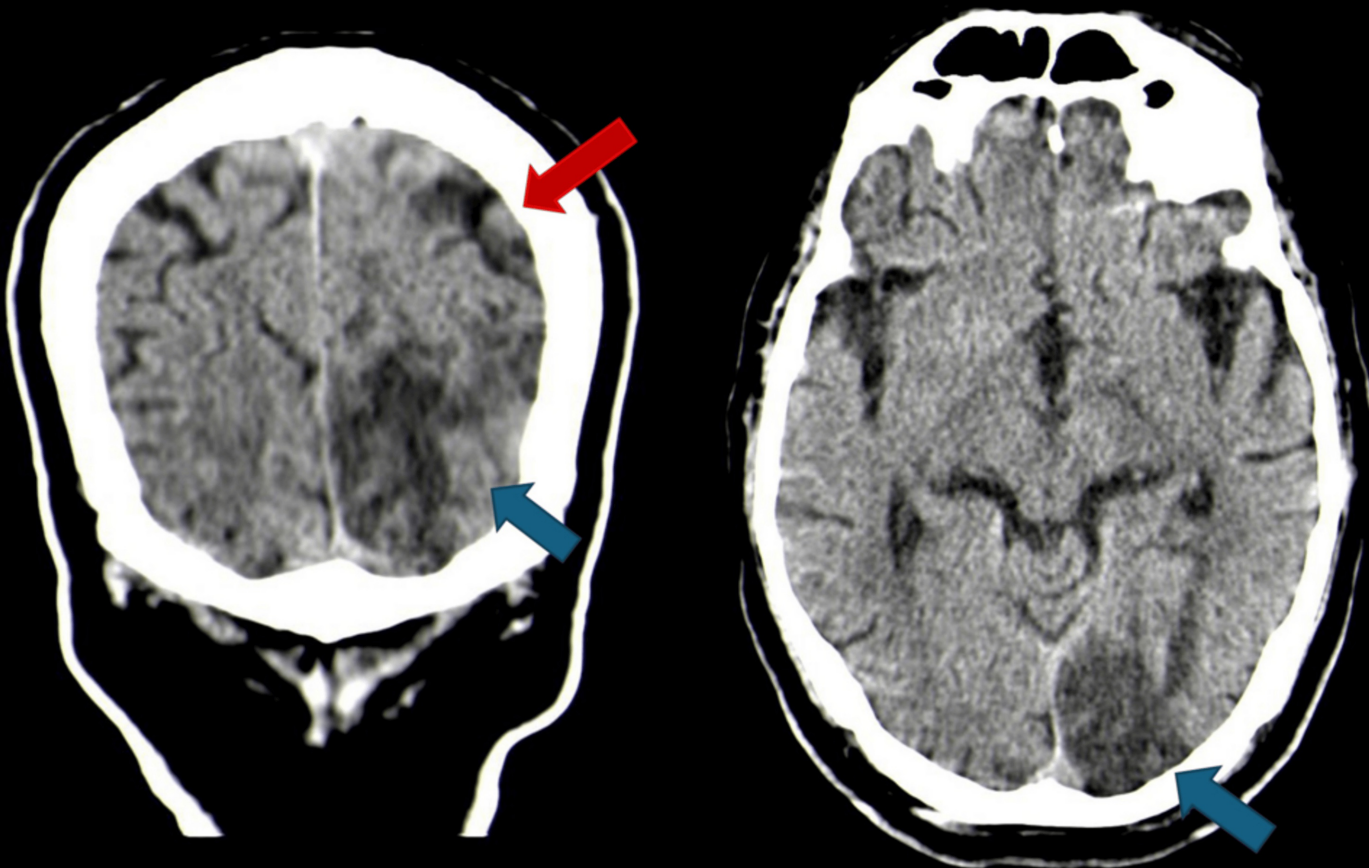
Cranial CT (coronal plane on the left and axial plane on the right): the blue arrow indicates an occipital infarction, while the red arrow highlights a small cortical parietal infarction in a different arterial territory.
